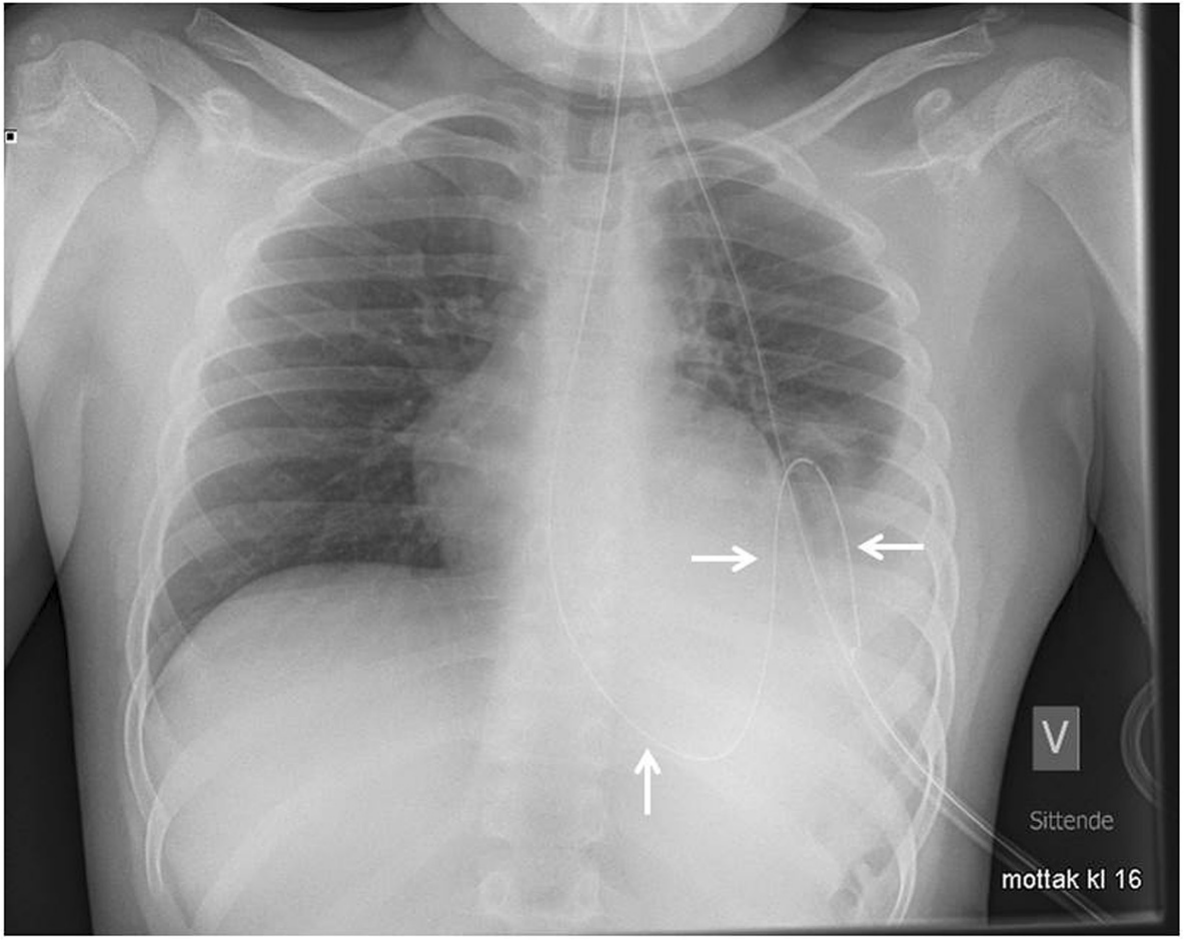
Chest x-ray after gastric decompression. Note the normalization of the mediastinal shift and the gastric tube as it curves back into the left hemithorax (arrows) confirming the intrathoracic position of the stomach
